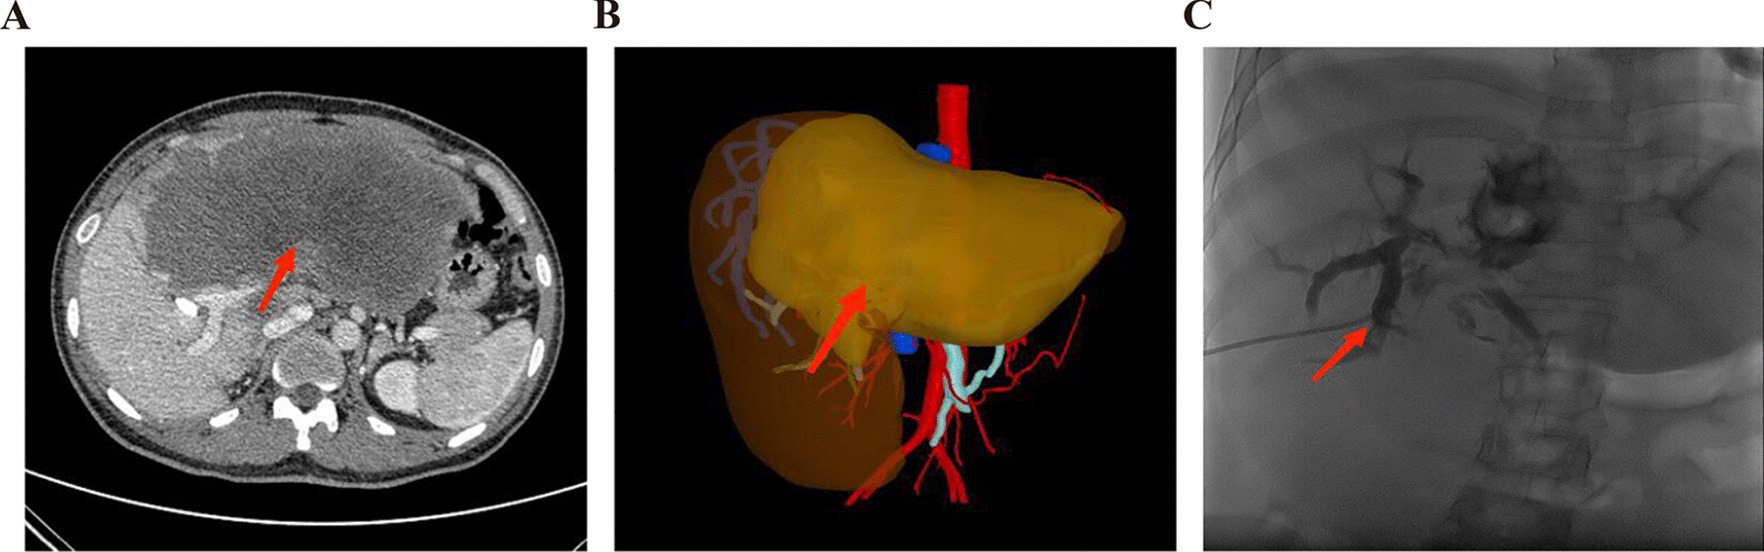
Representative preoperative images of PVE. A typical CT imaging before PVE; B digital 3D reconstruction before PVE (FLRV/TLV < 30%); C PTCD puncture and right posterior lobe biliary drainage
